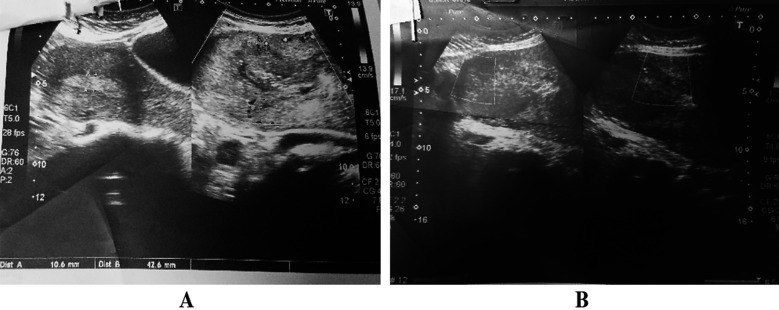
Doppler ultrasound showing bicornuate uterus (a) with placental invasion within myometrium(b).
